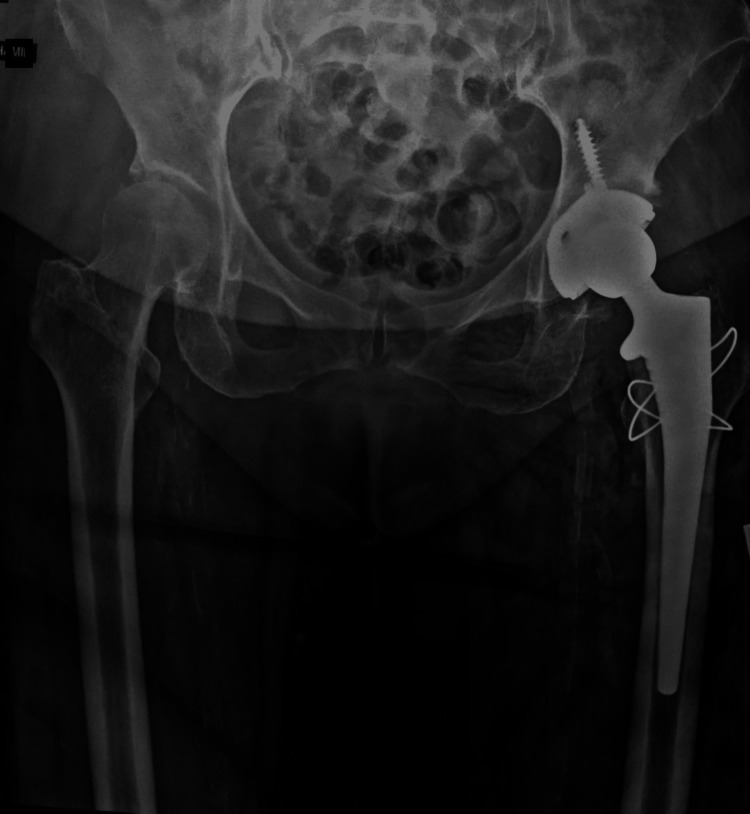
Postoperative X-ray of patient 3 with iatrogenic greater tuberosity fracture and managed with tension band wiring.
